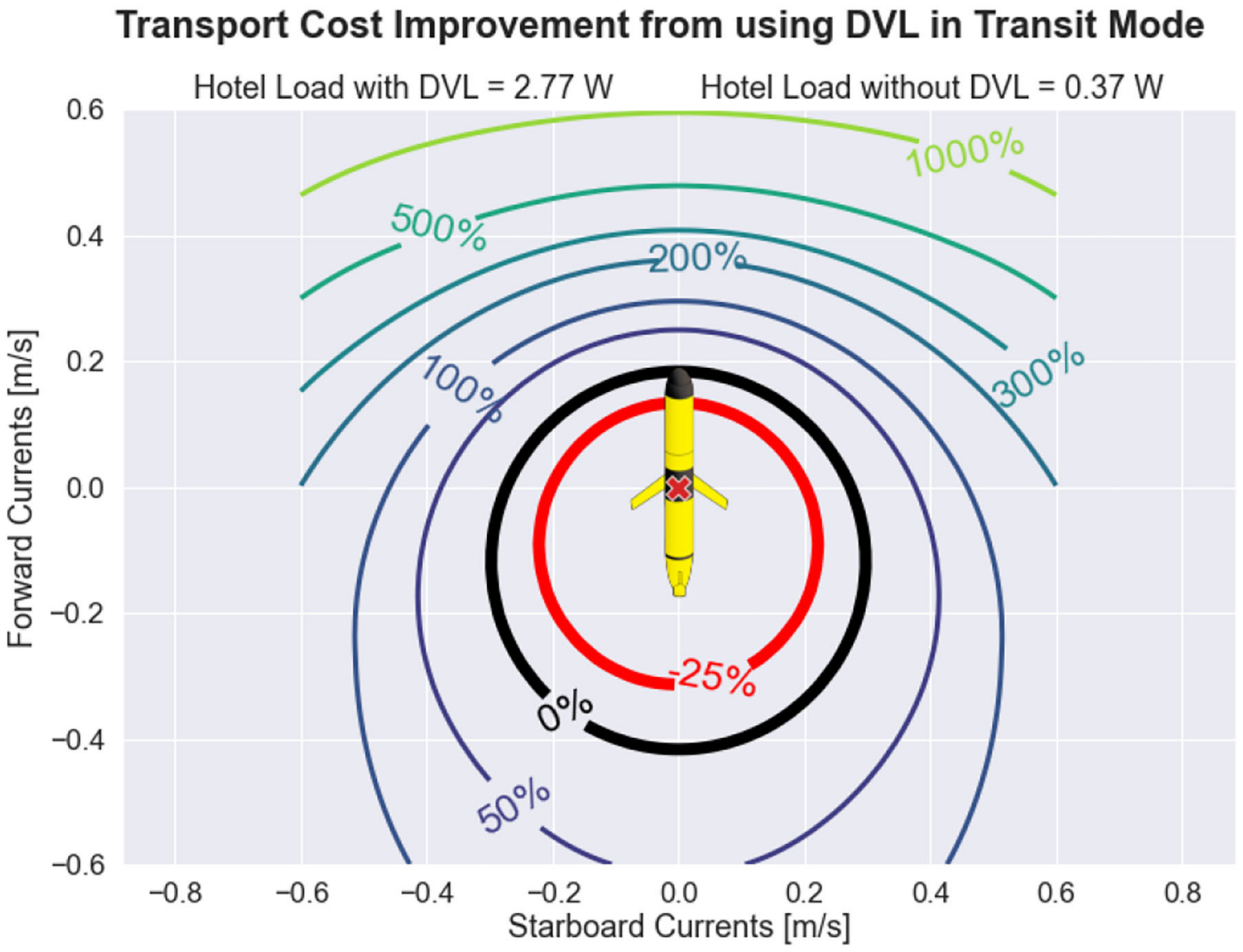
Transport cost improvement obtained by operating the Doppler velocity log (DVL) while transiting. By operating the DVL during transit, the autonomous underwater glider (AUG) can perform adaptive velocity control (AVC), which includes active steering to avoid cross-track and long-track drift caused by ocean currents and active throttling to take advantage of environmental conditions. The downside of operating the DVL is that the glider hotel load is increased. Thus, for ocean currents of small magnitudes, transport cost is actually worsened when the DVL is operated during transit, but for ocean currents of moderate magnitudes, transport cost can be substantially improved.
